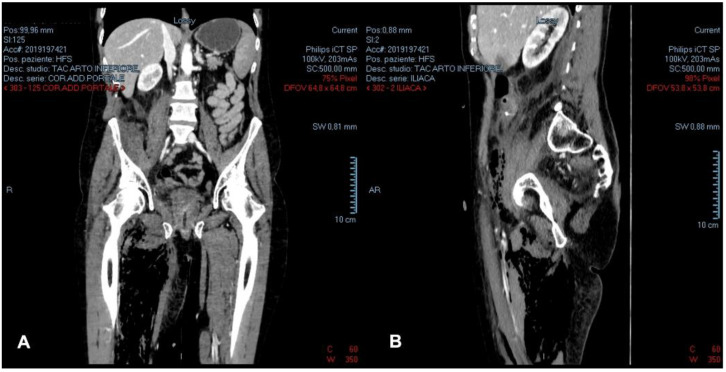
Pre-operative contrast-enhanced portal phase CT-scan of the abdomen and upper thighs: (A) coronal plane; (B) sagittal plane.
